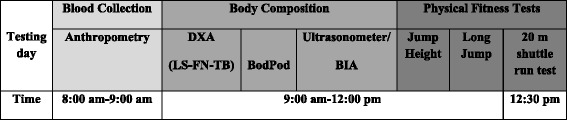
Order of the measurements for each testing day. DXA, dual energy x-ray absorptiometry; LS, lumbar spine; FN, femoral neck; TB, total body; BIA, bioelectrical impedance analysis.
